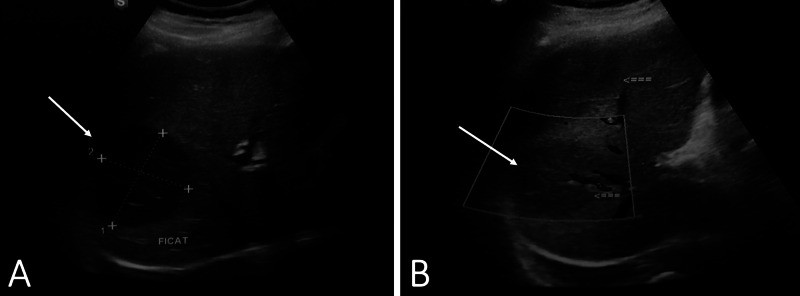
Ultrasonography of the liver showing masses (arrow) in the right (A) and left hepatic lobes (B).
